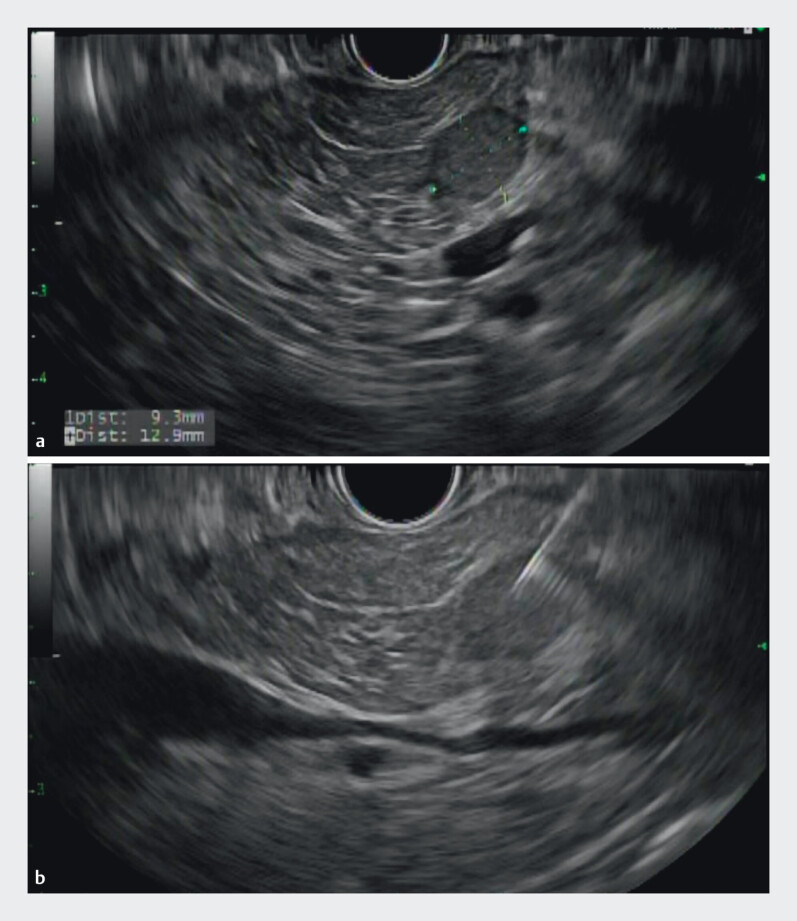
Endoscopic ultrasound (EUS) image showing:
